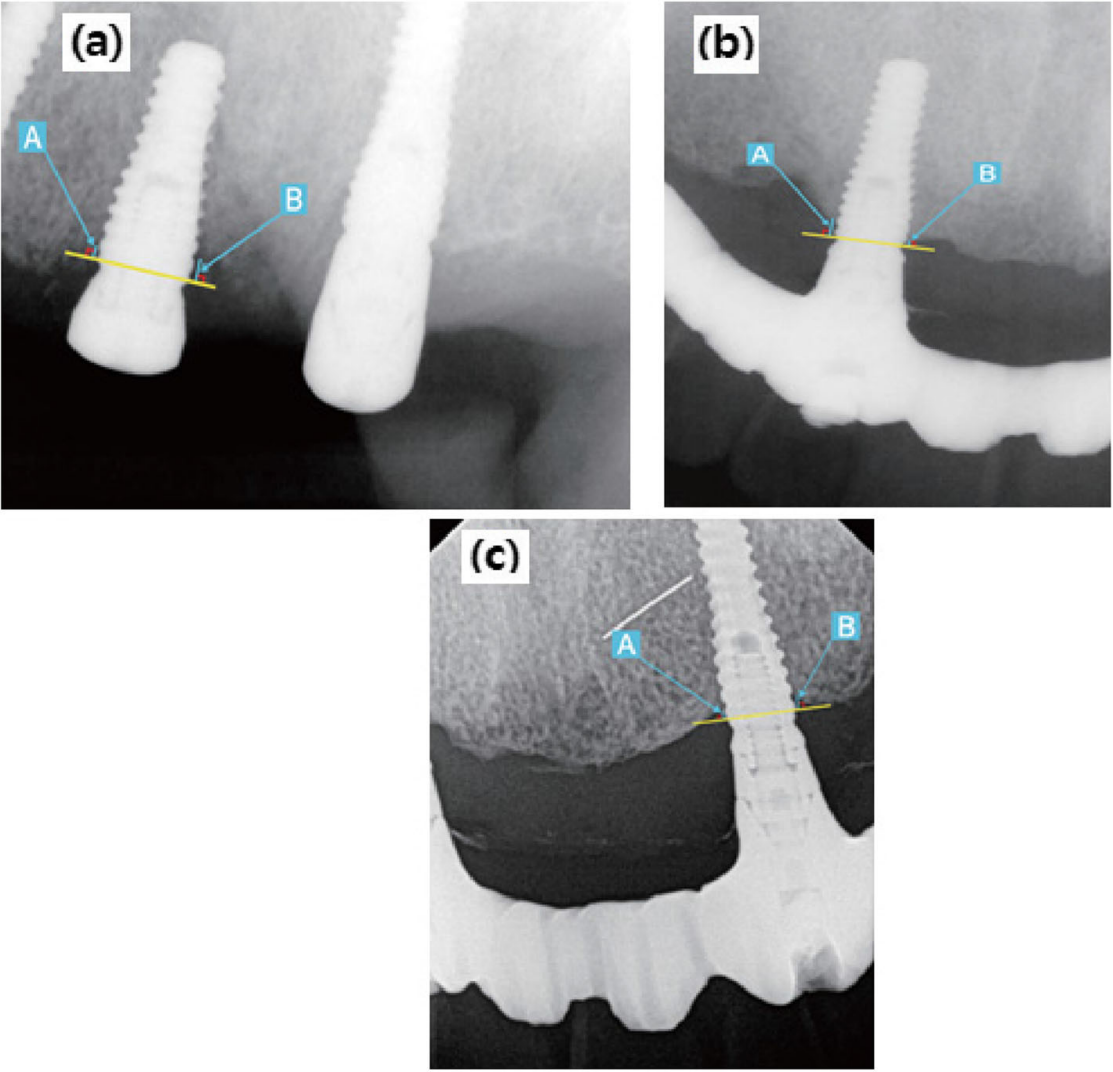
Landmarks of the radiographic measurements. a Immediately after surgery periapical view. b Periapical view 1 year after prosthetic loading. c Final periapical radiograph. [A point: linear distance from the implant shoulder to the contact point of the implant and bone (mesial surface), B point: linear distance from the implant shoulder to the contact point of the implant and bone (distal surface). The average value of A point and B point was set as the marginal bone loss amount.]
